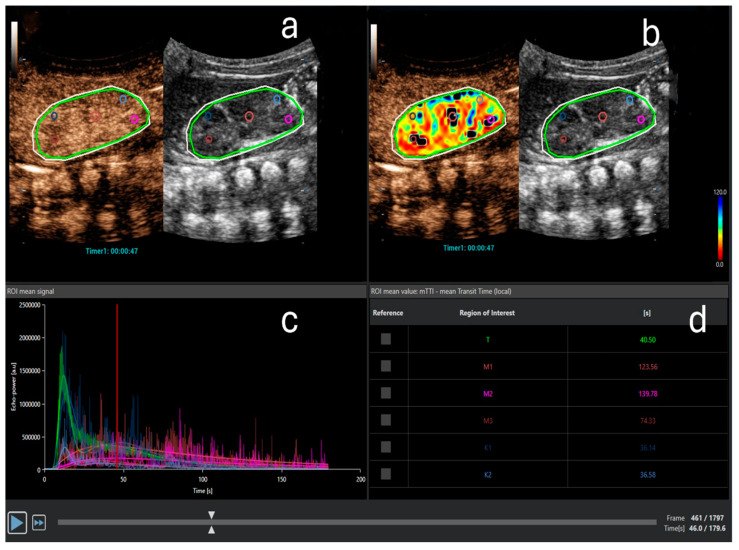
Contrast-enhanced ultrasound quantitative analysis. (a) Three regions of interest (ROIs) in the medulla (pink, purple, and red) and two ROIs in the cortex (light and dark blue) are designated. The light green oval ROI represents the entire kidney. The outer white ROI represents the area where analysis is performed. (b) Perfusion map for the parameter mean transit time (mTT) with selected ROIs. (c) Time-intensity curve (TIC): echo power in arbitrary units on the ordinate and time on the abscissa. Different curves represent the anatomical regions, marked with the same color as the ROIs in (a,b). Note the typical shape of the curve after bolus-contrast administration. (d) The quantitative analysis for mTT in seconds for the entire kidney (t), cortex (K1, K2), and medulla (M1–M3). mTT is the time to 50% of peak signal intensity and is prolonged in the medulla compared to the cortex due to the physiological renal perfusion, where the cortex receives most of the blood volume earlier than the medulla.
